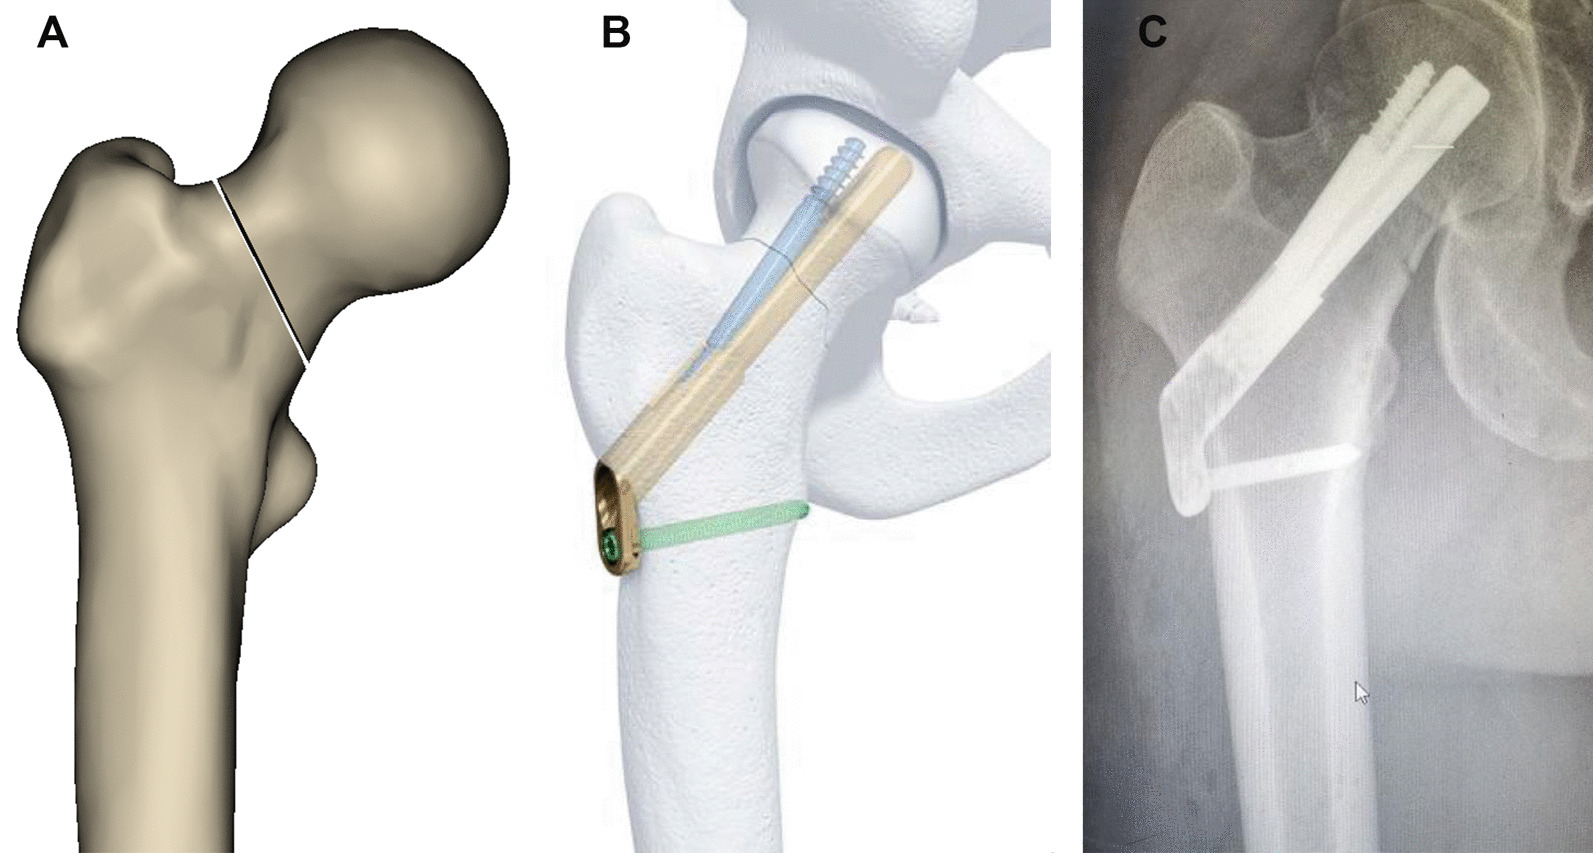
Femoral neck fracture model (A), internal fixation model of the new internal fixation femoral neck system (B), and X-ray visualization of FNS for femoral neck fracture (C)
